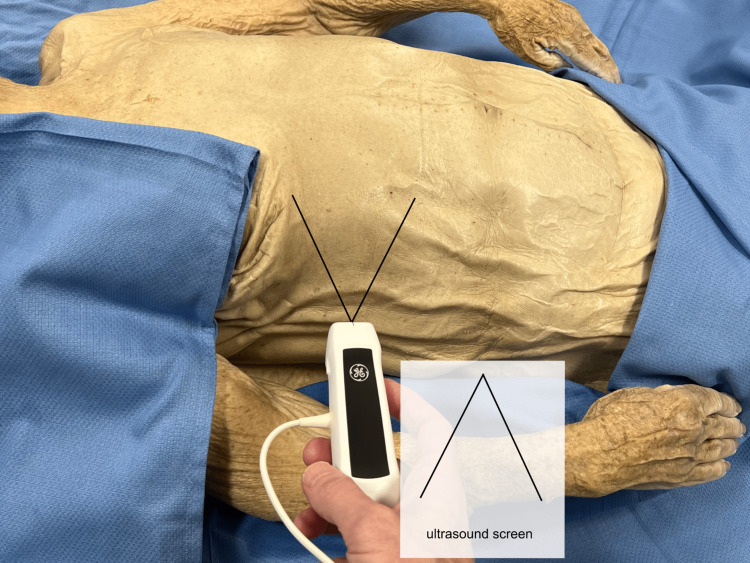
Right upper quadrant probe The perspective from the right upper quadrant would again need to be viewed as a reflection since the image on the ultrasound screen would be like a mirror image of the gross anatomy view.
